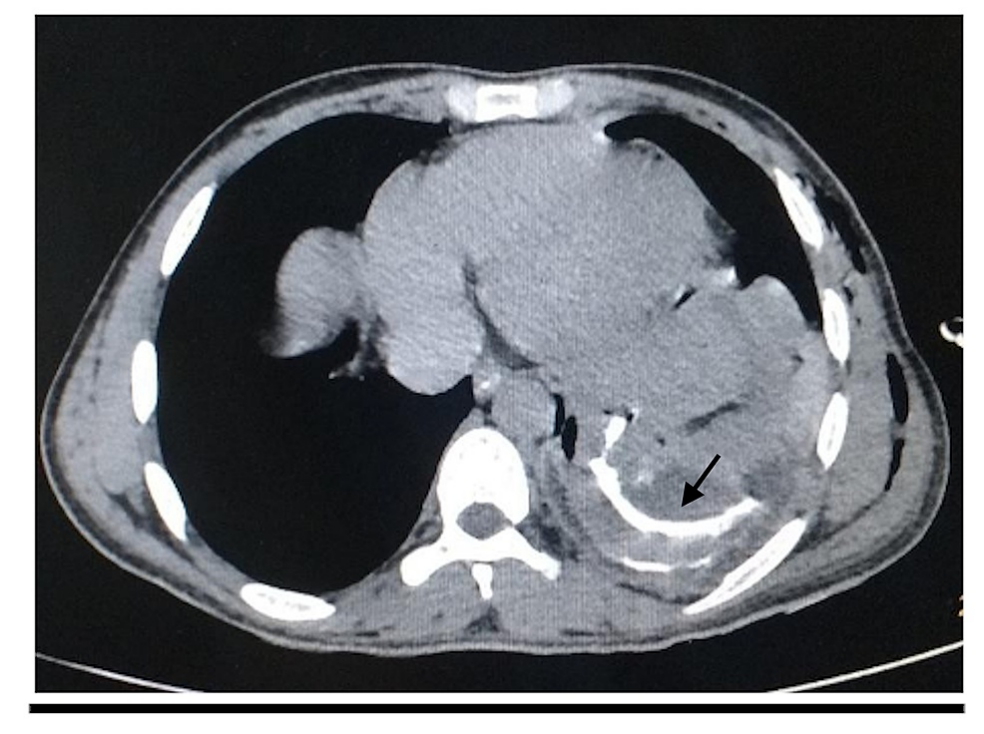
The CT scan done at the time of presentation The image shows herniated abdominal viscera with oral contrast in the left hemithorax (black arrow) CT: computed tomography
